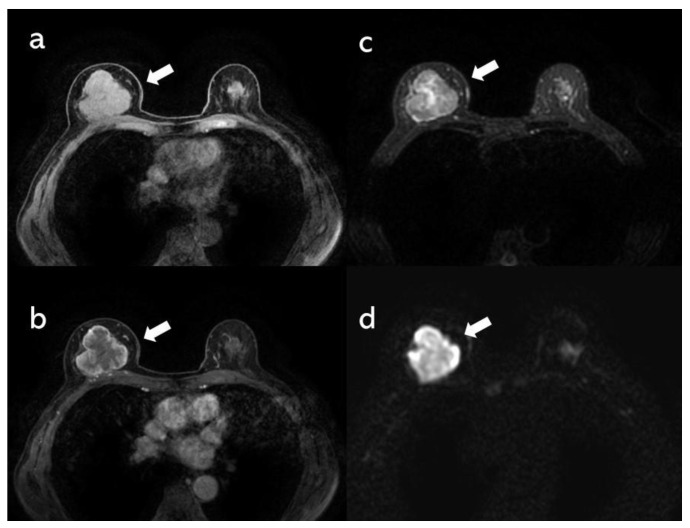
Magnetic resonance imaging shows a lobulated mass on the T1-weighted image (TWI) (a: arrow) with strong contrast mainly seen on the periphery on contrast-enhanced T1WI (b: arrow), a high signal in the center on T2WI (c: arrow) and a high signal in the peripheral on diffusion-weighted images (d: arrow).
